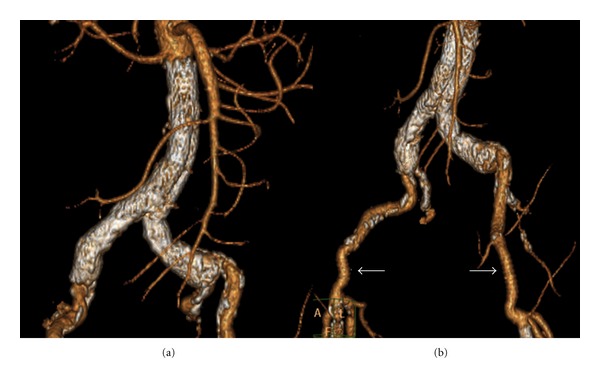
Six-month postprocedural computed tomography angiography revealing (a) the successful deployment of the aortic graft and (b) the patent femoral-femoral PTFE grafts (white arrows).
